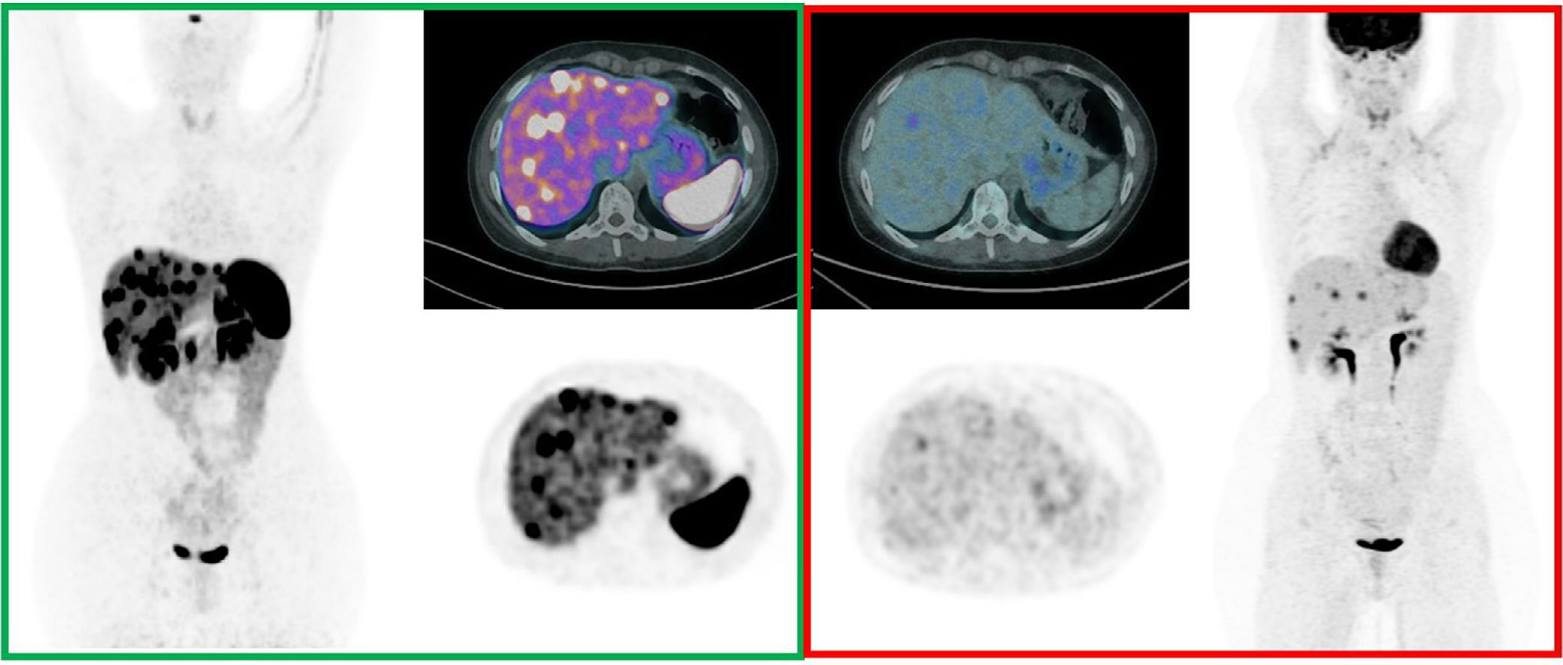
[^68^Ga]Ga‐DOTANOC (green box) and [^18^F]F‐FDG PET/CT (red box) images, acquired 1 day apart, in a 27 years old female patient. Indication was restaging after chemotherapy (CAPTEM) of a pancreatic NET G3 (Ki‐67: 28%). [^68^Ga]Ga‐DOTANOC PET/CT detected multiple focal lesions of liver uptake (green box), only some of them also demonstrated [^18^F]F‐FDG PET/CT uptake (matched findings) (red box).
